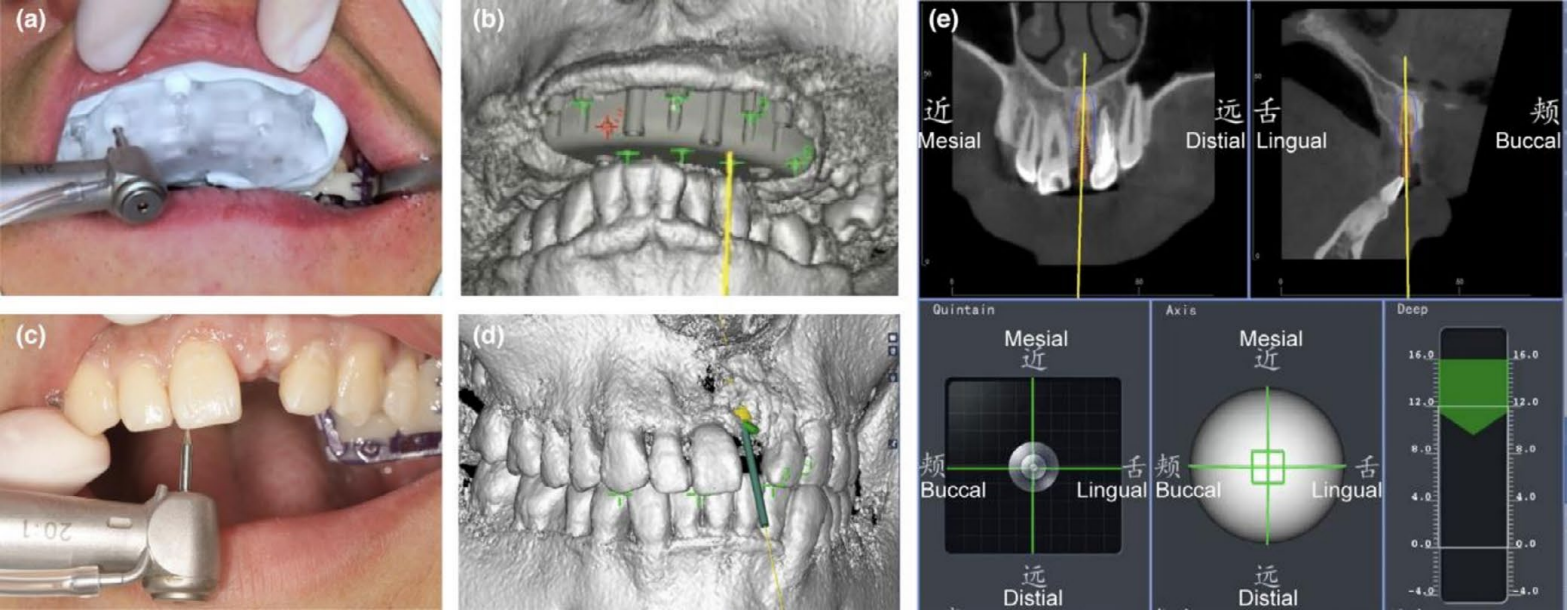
Surgical procedures for the M‐FBR and F‐PBR during d‐CAIS. The custom splint which was re‐positioned onto the patient's dental arch (a, b). The radiopaque fiducial markers on the splint were paired with the corresponding high‐density points shown in the CBCT to achieve marker‐based registration. F‐PBR was accomplished by pairing the selected cusps or fossae with the corresponding anatomic structures shown in the CBCT (c, d). The drilling and implant insertion procedures were guided by the navigation system (e) [27].
